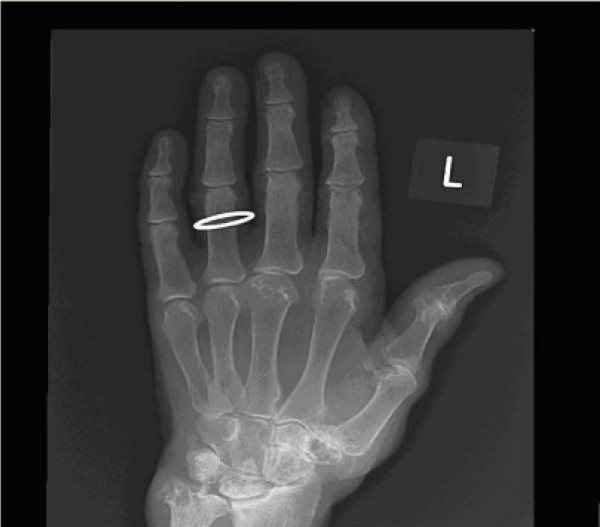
Plain x-ray appearance of the degenerative changes in the first carpometacarpal joint.
